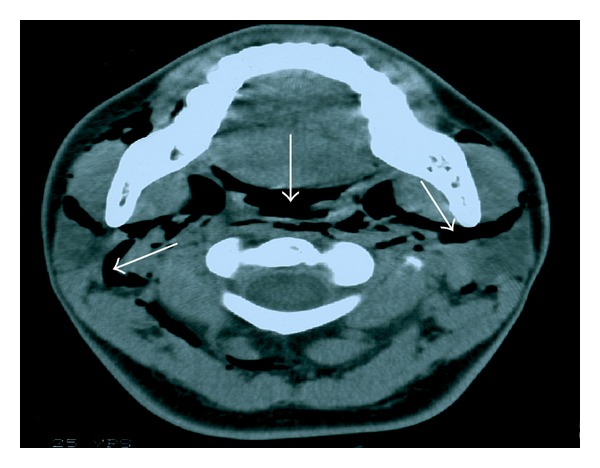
Axial computed tomography of the neck at the level of the inferior alveolar ridge reveals subcutaneous emphysema. The arrows indicate the presence of air in the neck.
